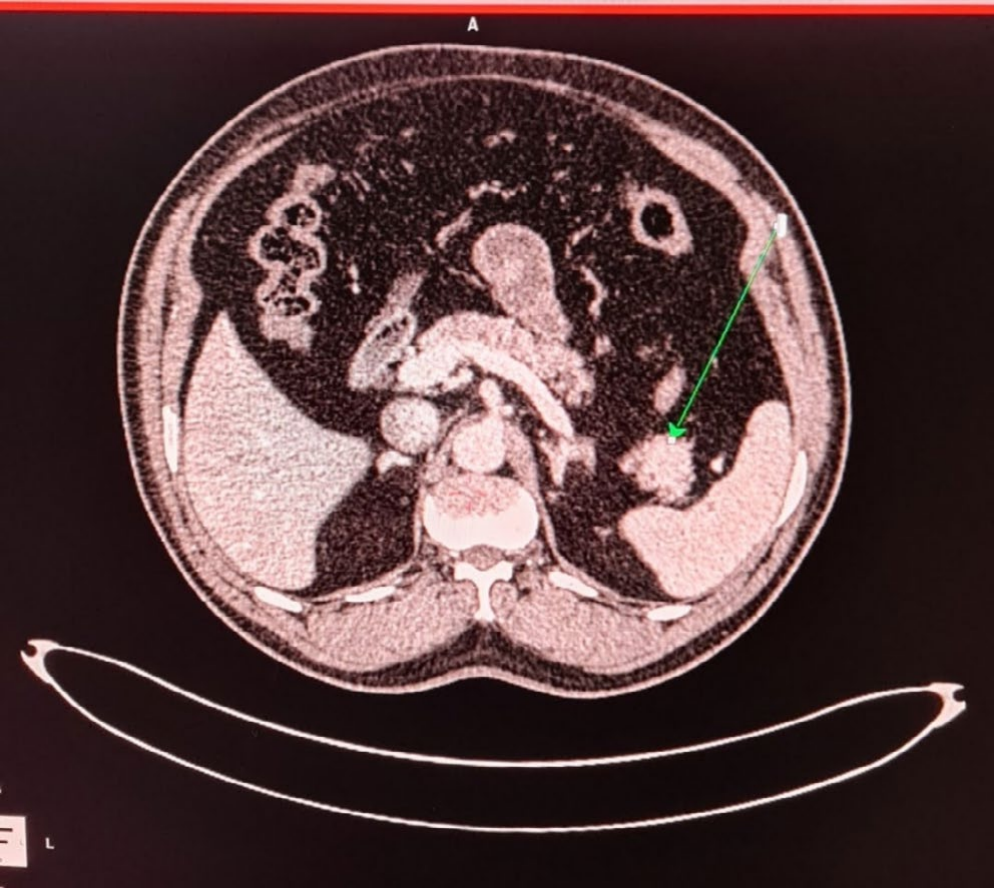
Contrast‐enhanced computed tomography of the abdomen. Arrow showing solid, slightly arterial phase hyper‐enhancing nodules within the tail of the pancreas with a small inferior exophytic component abutting branches of the splenic vein and artery superiorly.
